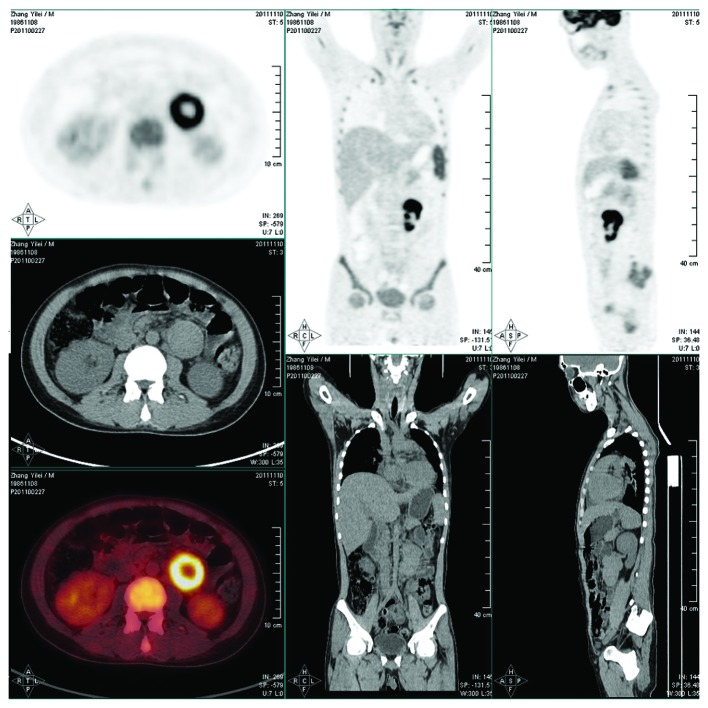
Positron emission tomography/computed tomography (PET/CT) revealed the metabolism of fludeoxyglucose (FDG) increased abnormally.
